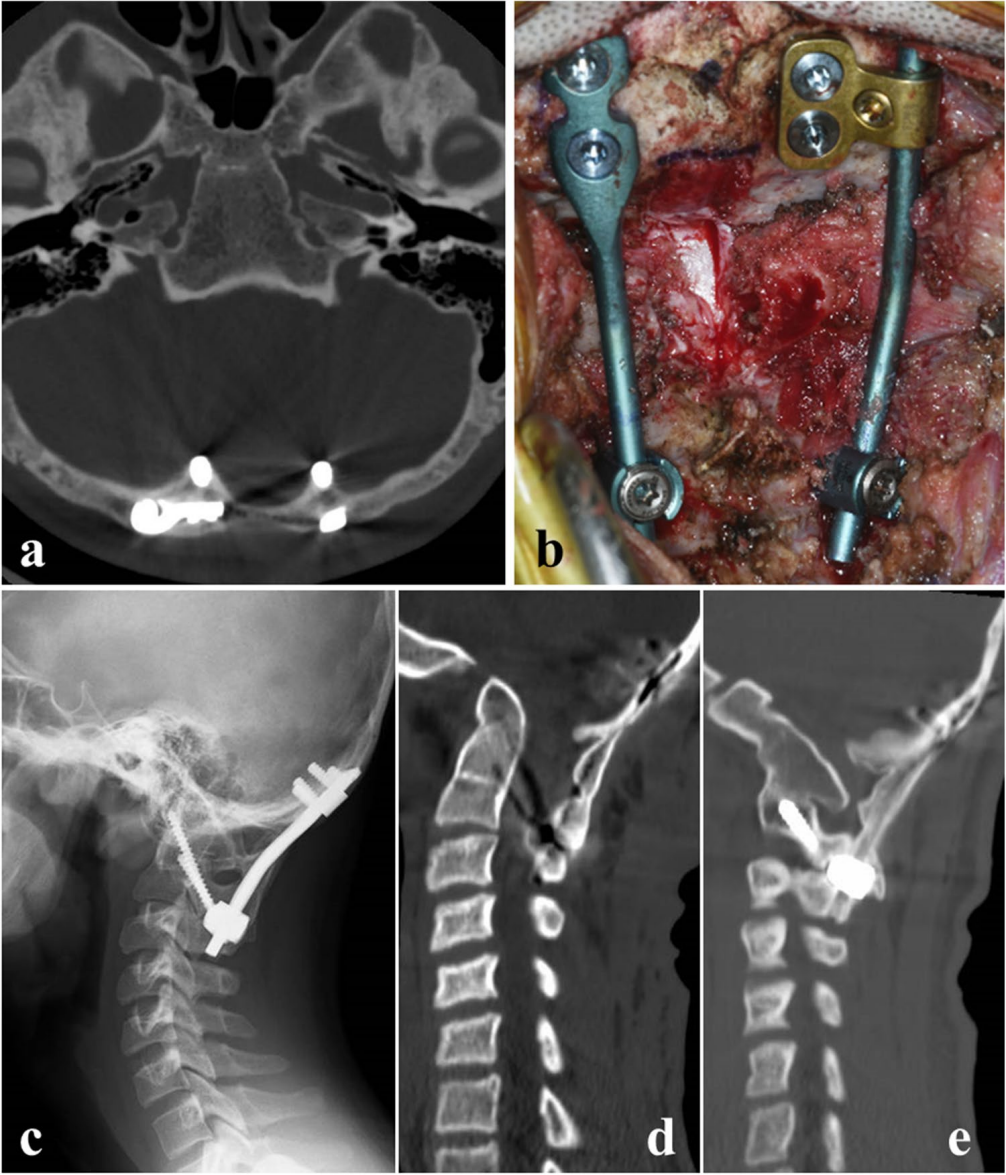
Surgery for upper cervical myelopathy caused by a congenital atlanto-axial dislocation complicated by os odontoidum. a Postoperative axial computed tomography demonstrating occipital screws placed bilaterally into the bifurcated internal occipital crests. b Intraoperative photograph showing decompression of the foramen magnum and posterior arch of C1 with occipital plates separately placed bilaterally. c Lateral radiograph of the cervical spine 2 years after surgery. d, e Sagittal reconstruction computed tomography 2 years after surgery showing solid fusion of the O-C2 segment. Note that the os odontoideum is fused to the base of the axis
